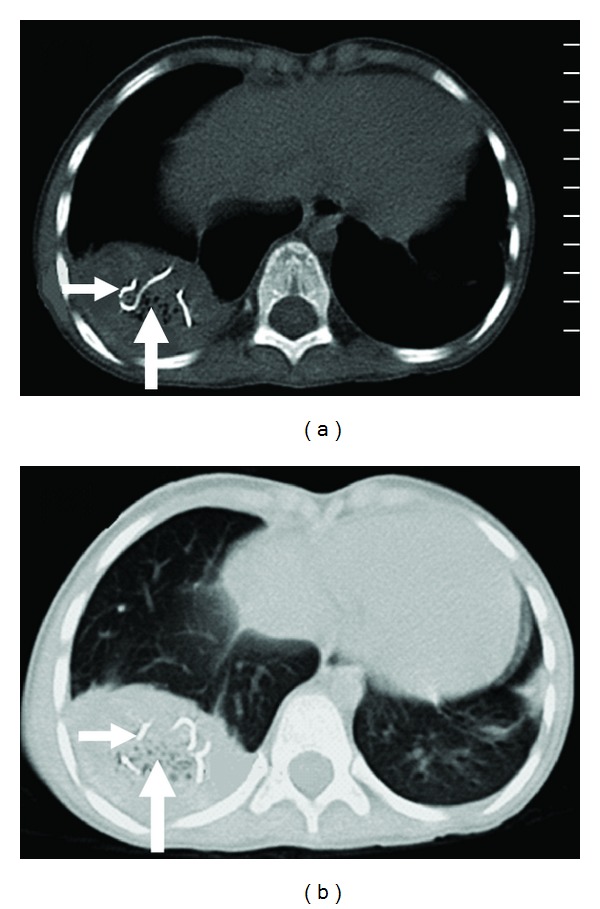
Un-enhanced CT scan section of the thorax, in bone window (a) and lung window setting (b) showing a well-defined, hypodense, pleural based mass in the right lower hemithorax. The whorl-like structure is representing the sponge itself, which is having air droplets (Large Arrows) and a thin, coiled structure of high density in core of the lesion, representing the radio-opaque markers (Small Arrows).
